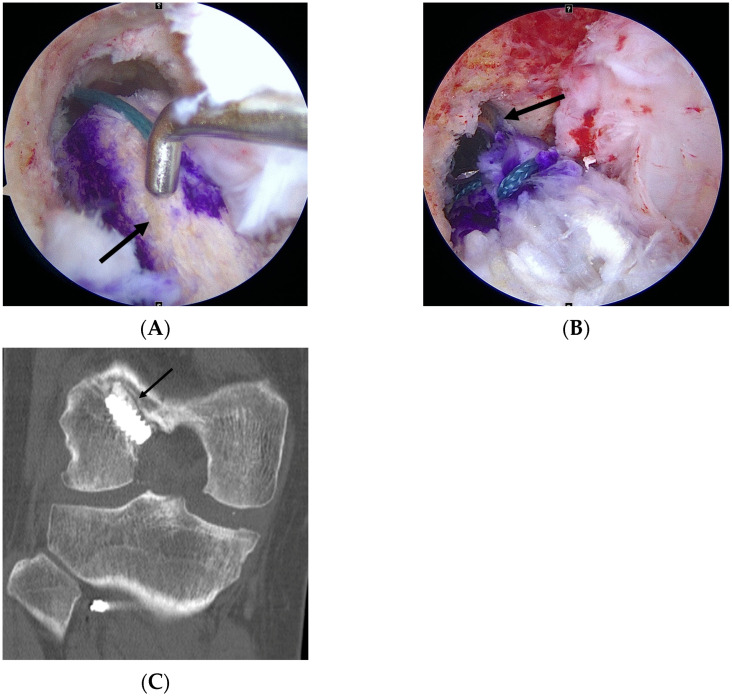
Femoral tunnel bone–block fixation. (A) The bone block (black arrow) is carefully introduced into the femoral tunnel, with a probe used to guide insertion and maintain proper orientation. (B) Intraoperative view showing the bone block fully seated within the tunnel and stabilized by a metallic interference screw (black arrow). (C) Postoperative coronal CT image demonstrating secure fixation of the bone block (black arrow) with the metallic interference screw within the femoral tunnel.
